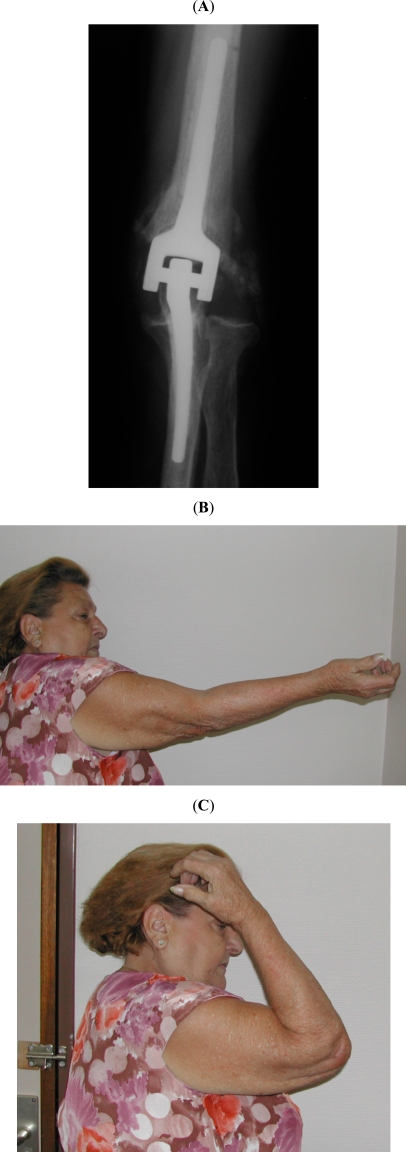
Postoperative radiograph (A) and final range of motion (B and C) after elbow arthroplasty for an acute distal humerus fracture in an elderly female patient.
